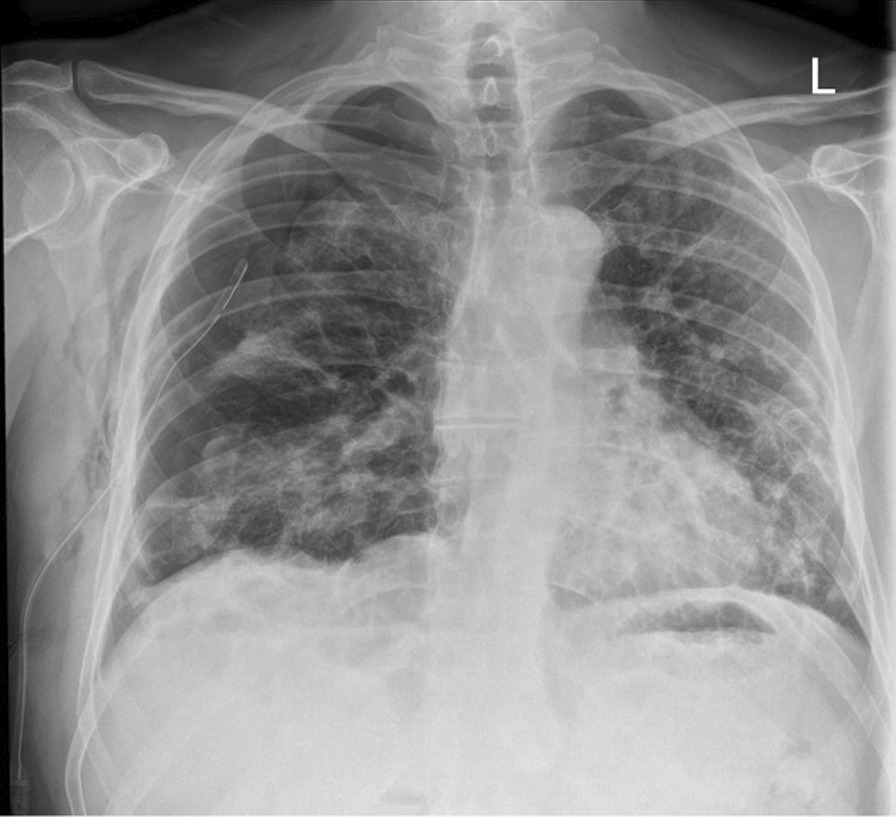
Chest x-ray of tension pneumothorax following chest drain insertion: Partial resolution of tension pneumothorax
